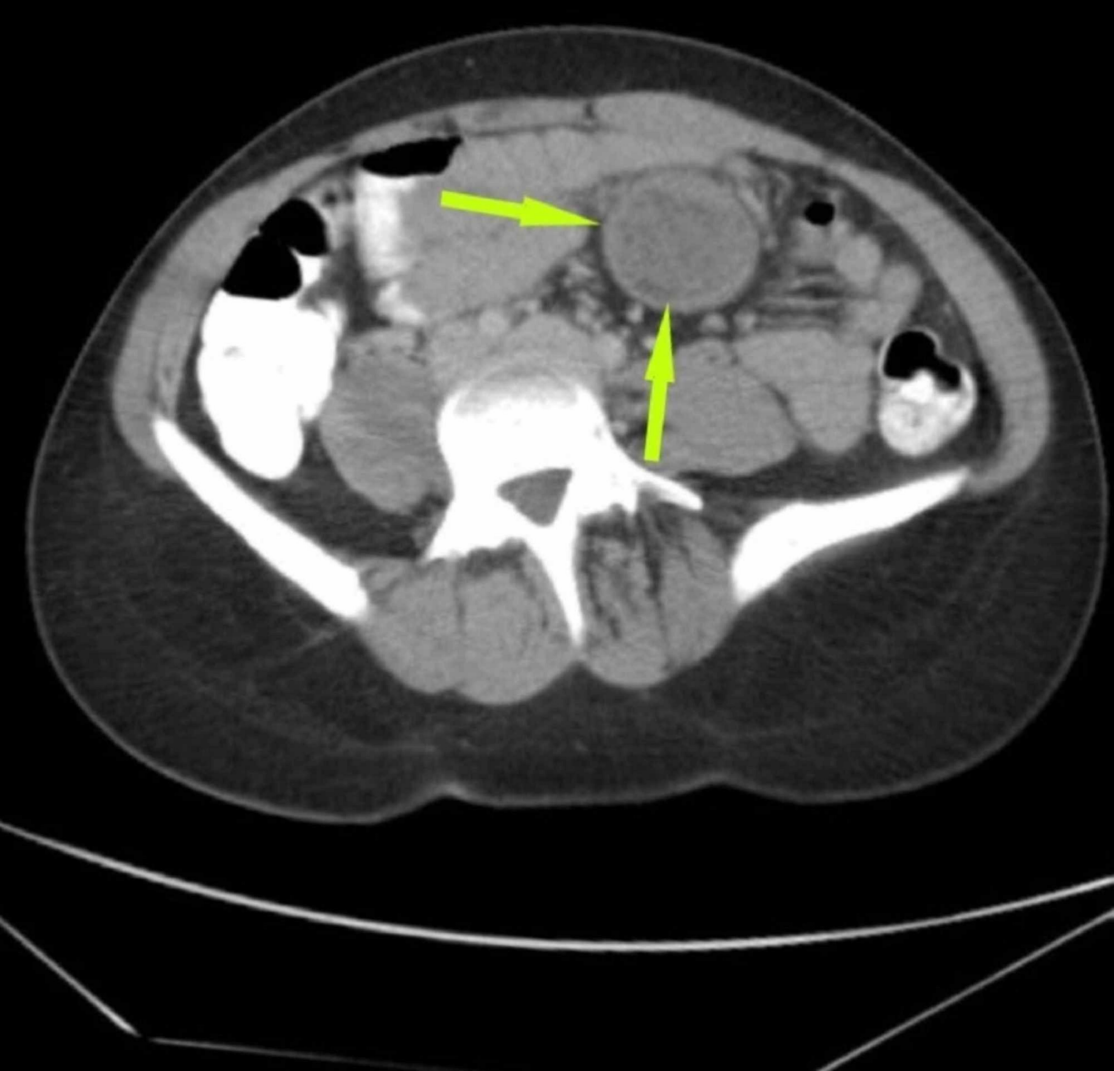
Computed tomography scan of the abdomen showing the mesenteric cyst (yellow arrows)
